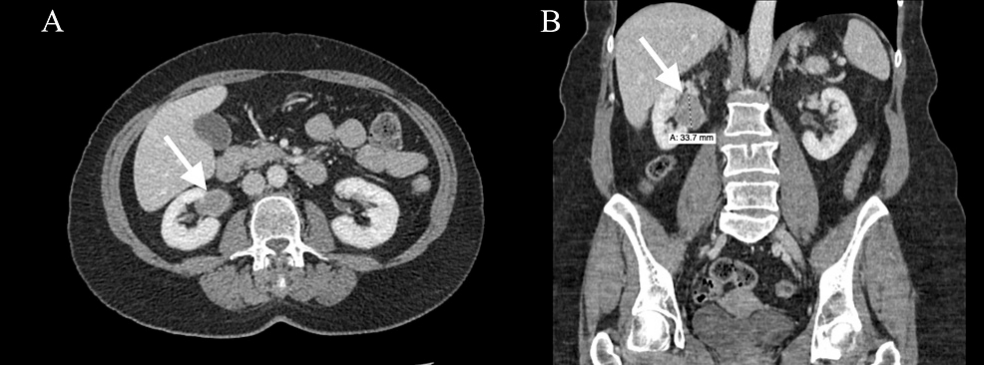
Representative CT of right renal mass (A) Axial and (B) coronal views showing a 3.4 cm tumor abutting the renal pelvis (solid white arrow).
